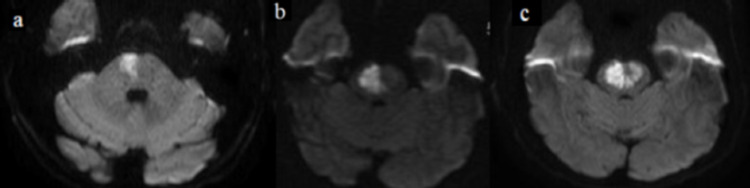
MRI images. a. Diffusion-weighted increased signal on the right pontine side. b. Right-sided signal expansion. c. New diffusion-weighted increased signal on the left side, similar to the “heart appearance” sign.
